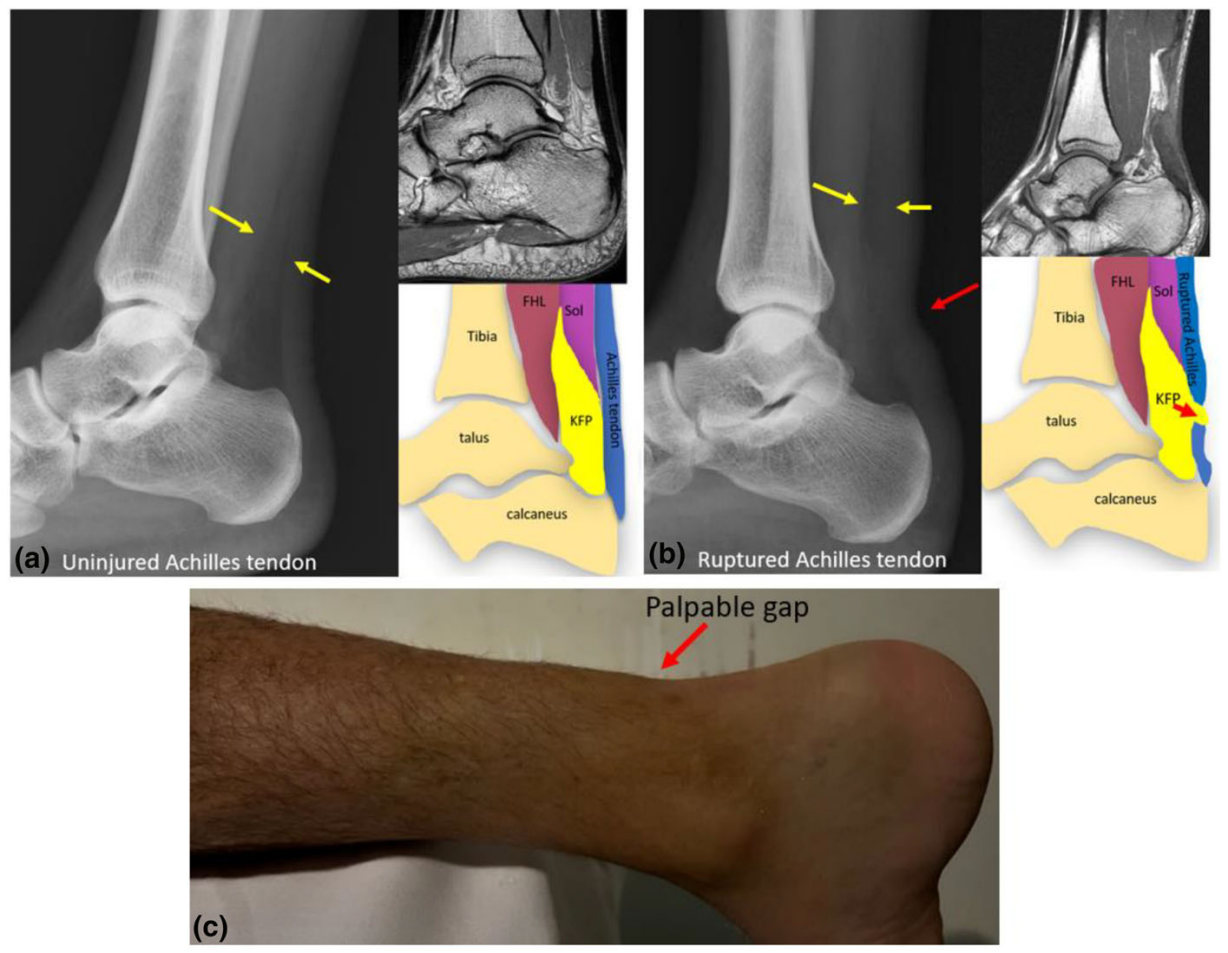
Radiographs and magnetic resonance (MR) images demonstrating an intact and ruptured Achilles tendon. (a) Intact Achilles tendon. A clearly defined Kager's triangle is observed as a clearly defined area of low density (yellow arrows) on a lateral radiograph. On a sagittal MRI, Kager's triangle is clearly defined. (b) When the Achilles tendon is ruptured, the margins of Kager's triangle, outlining the region of the pre‐Achilles fat pad become distorted on a plain lateral radiograph (yellow arrows). On a sagittal MR imaging, Kager's fat pad herniates into the gap between ruptured tendon ends. (c) Photograph of the palpable region of a ruptured Achilles tendon. FHL, flexor hallucis longus muscle; KFP, Kager's fat pad; Sol, soleus muscle.
